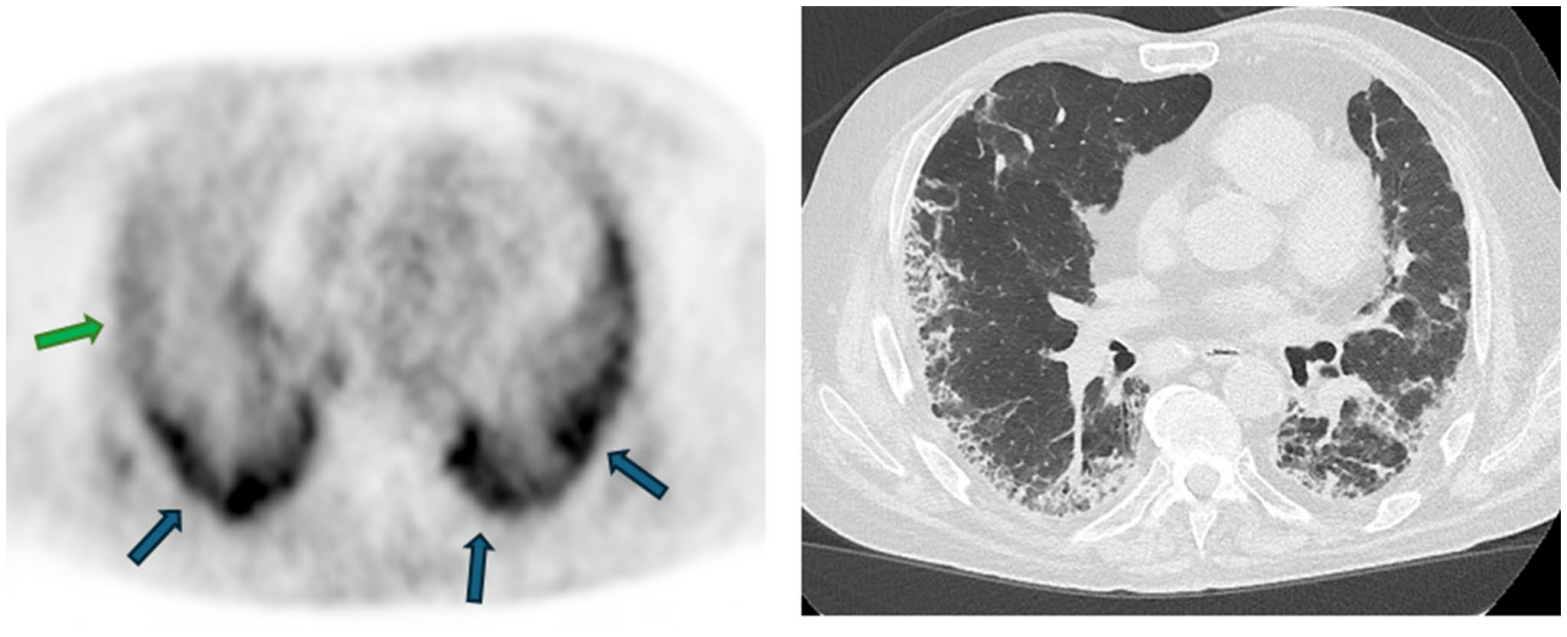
^68^Ga-FAPI uptake assessed by PET/CT in a patient with idiopathic pulmonary fibrosis and the corresponding high-resolution CT. An intense uptake of the radiotracer is observed in some subpleural lesions (blue arrows), whereas some present a weak uptake (green arrow), suggesting a lower fibrogenesis activity kindly provided by the Department of Nuclear Medicine of the Hôpital Universitaire de Bruxelles (HUB), Brussels, Belgium.
